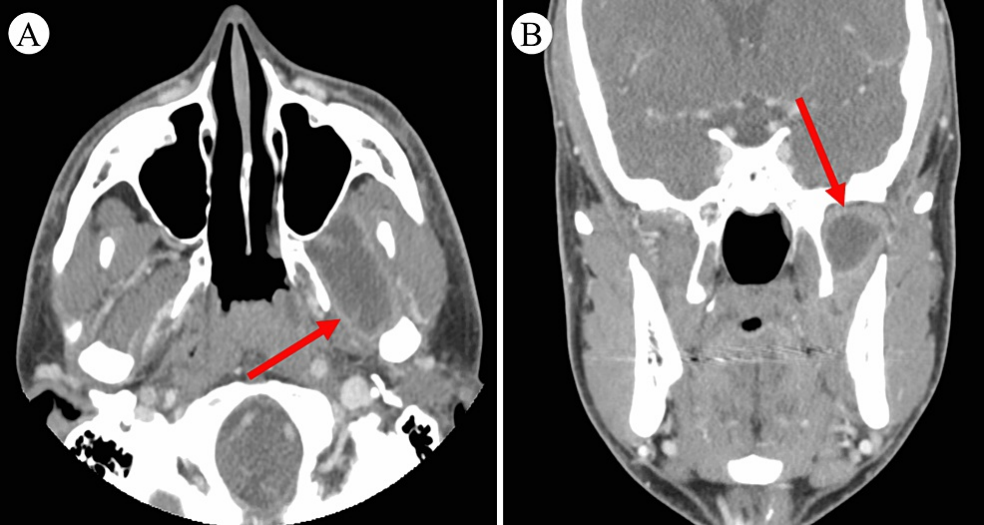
Maxillofacial CT demonstrating infratemporal fossa abscess of the lateral pterygoid (A) Axial CT of the abscess. (B) Coronal CT of the abscess.
